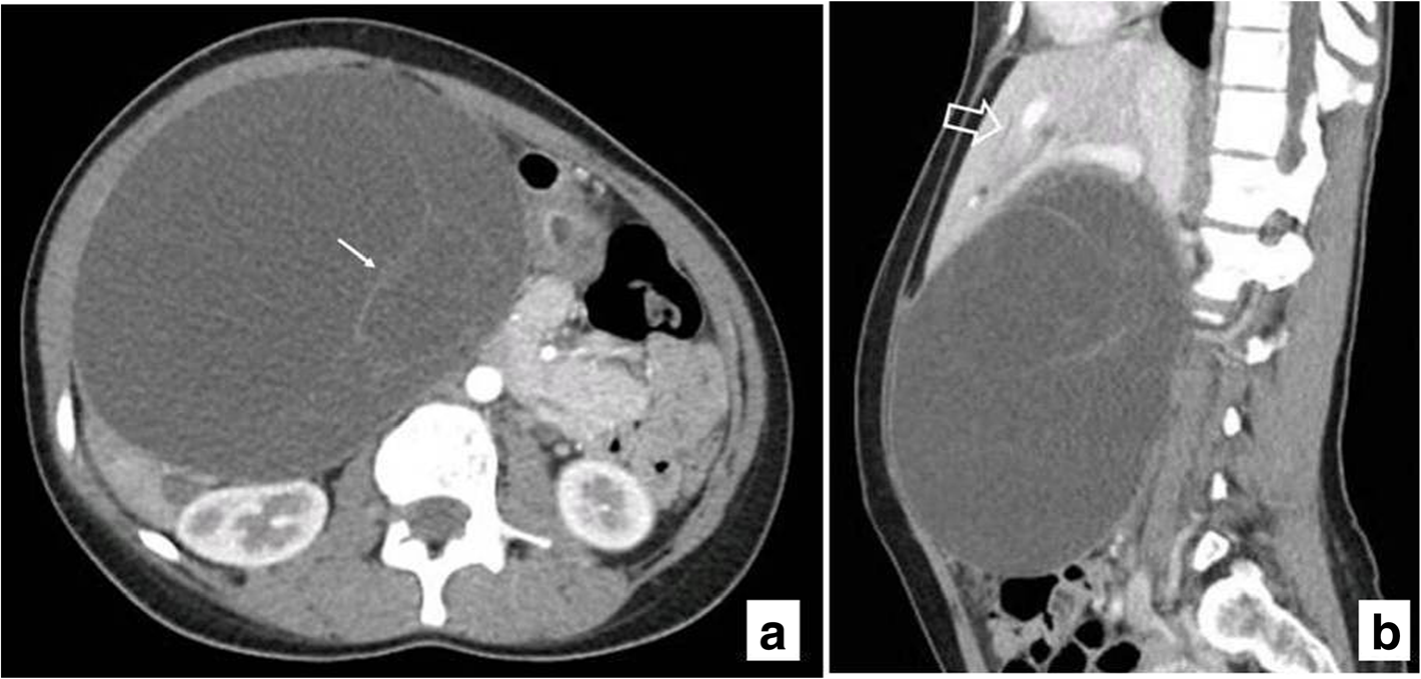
Axial and sagittal sections from computed tomography of the abdomen. a There is a large, well-circumscribed, multiloculated, intrahepatic cystic lesion. Fine internal septations without enhancing component are appreciated (*thin arrow*). b There is associated mass effect resulting in splaying of branches of portal vein and intrahepatic biliary dilatation (*thick arrow*)
